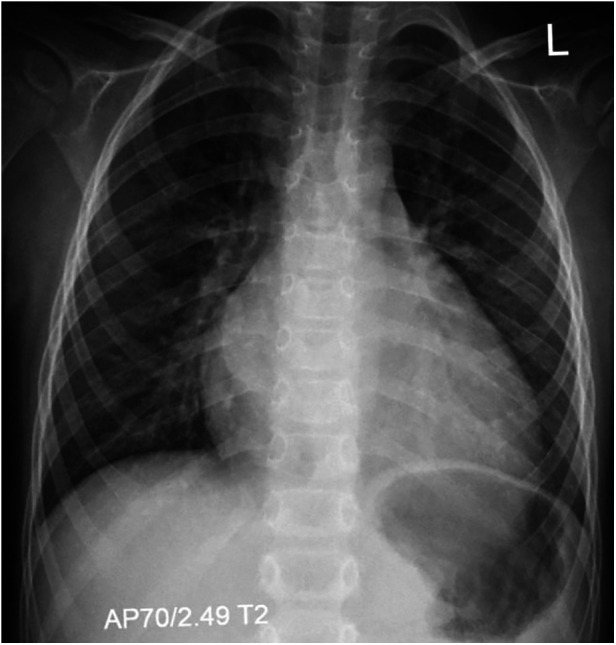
Chest x-ray (AP view) showing marked cardiomegaly consistent with dilated cardiomyopathy, without signs of pulmonary congestion.
